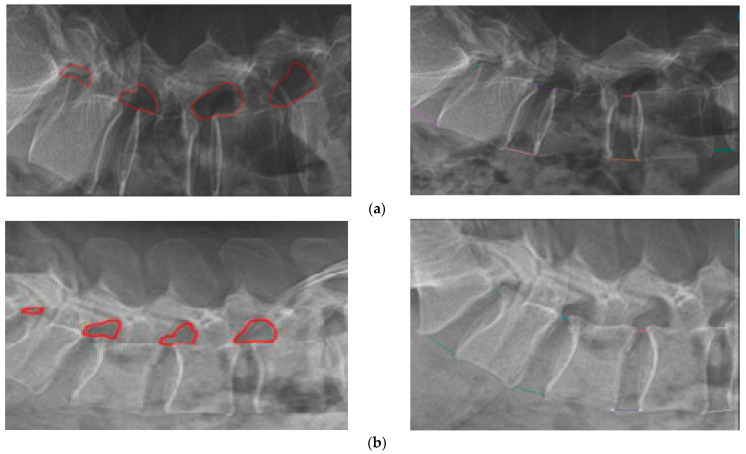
Image annotation of intervertebral foramen (IVF), posterior disc height (PDH), and anterior disc height (ADH) using lumbar X-ray images. Example of the image annotation and quantification of the IVF, PDH, and ADH in lumbar X-ray images. (a) The X-ray images of Volunteer A. (b) The X-ray images of Volunteer B.
